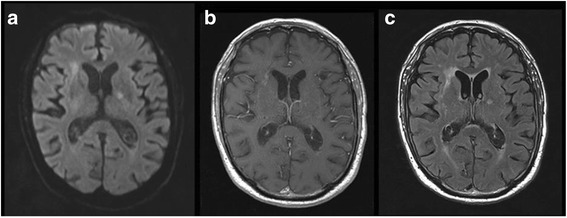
Brain magnetic resonance imaging at four weeks of treatment in axial multiple sequences. Decrease of the level of debris layering the occipital horns of the lateral ventricles in all sequences: axial diffusion b1000 (a), axial T1 gadolinium (b) and axial T2 fluid attenuated inversion recovery (FLAIR) gadolinium sequences (c). Clear decrease of the periventricular hyperintensities and ependymal enhancement in T1 with gadolinium (b) and T2 FLAIR gadolinium (c) sequences. Decrease of b1000 DWI hyperintensities. No apparition of new ischemic lesions
